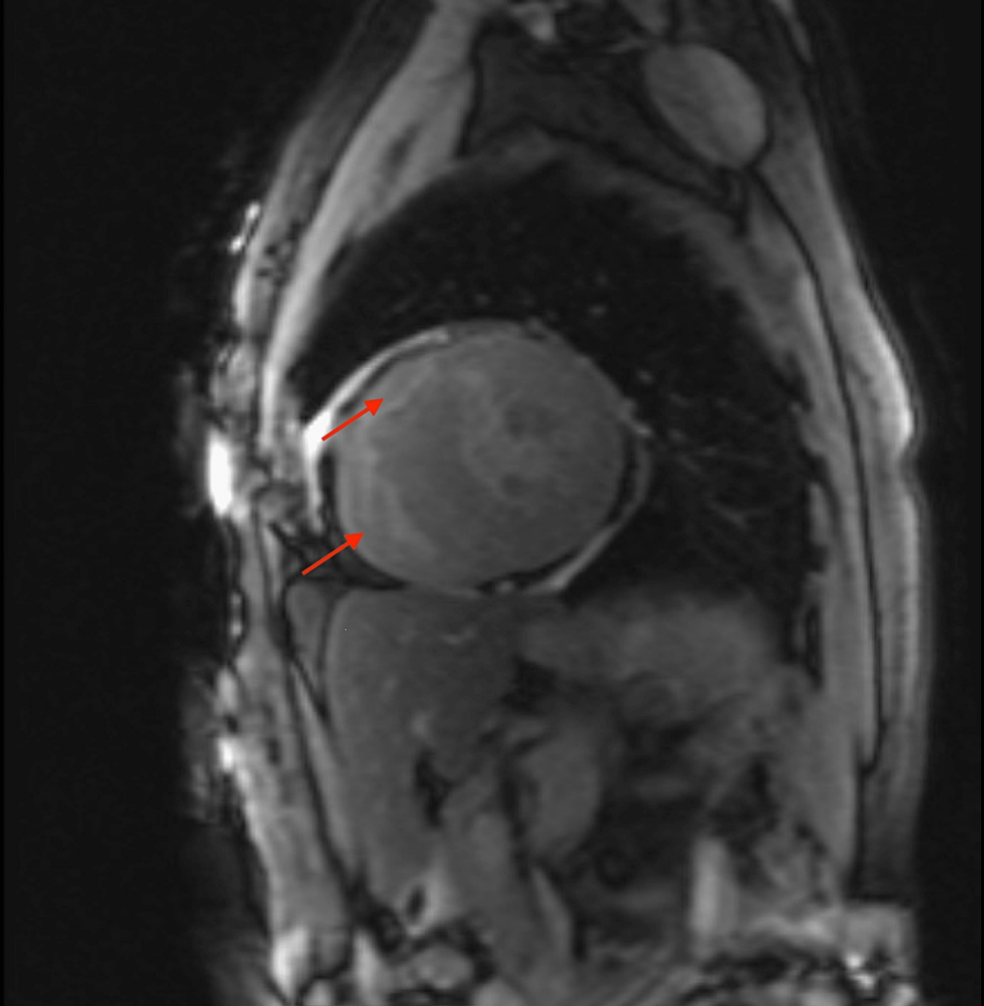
T1 Mapping on cMRI. Avid mid-myocardial LGE involving inferoseptal, anterior, and anterolateral segments (red arrows). LGE: late gadolinium enhancement, cMRI: cardiac magnetic resonance imaging.
